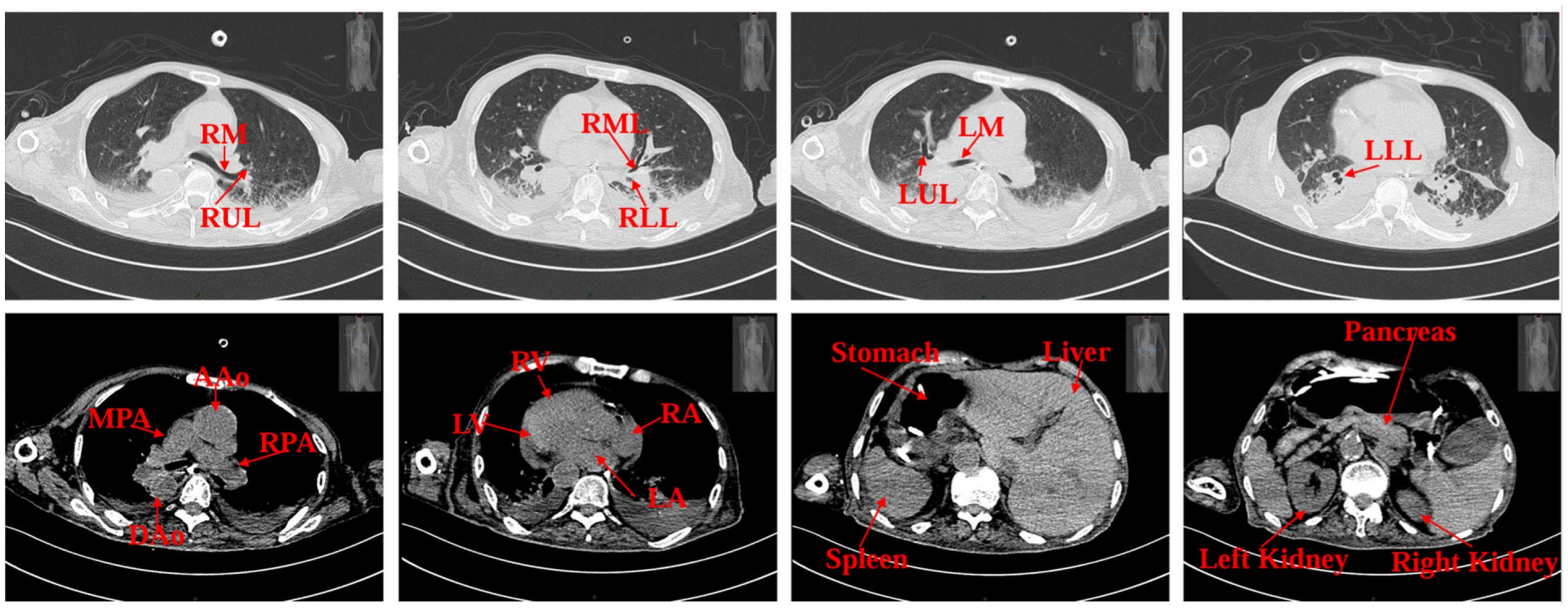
Patient’s CT scan images on admission. RM (right main bronchus), RUL (right upper lobe of lung), RML (right middle lobe of lung), RLL (right lower lobe of lung), LM (left main bronchus), LUL (left upper lobe of lung), LLL (left lower lobe of lung), MPA (main pulmonary artery), RPA (right pulmonary artery), AAo (ascending aorta), DAo (descending aorta), LV (left ventricle), LA (left atrium), RV (right ventricle), RA (right atrium).
